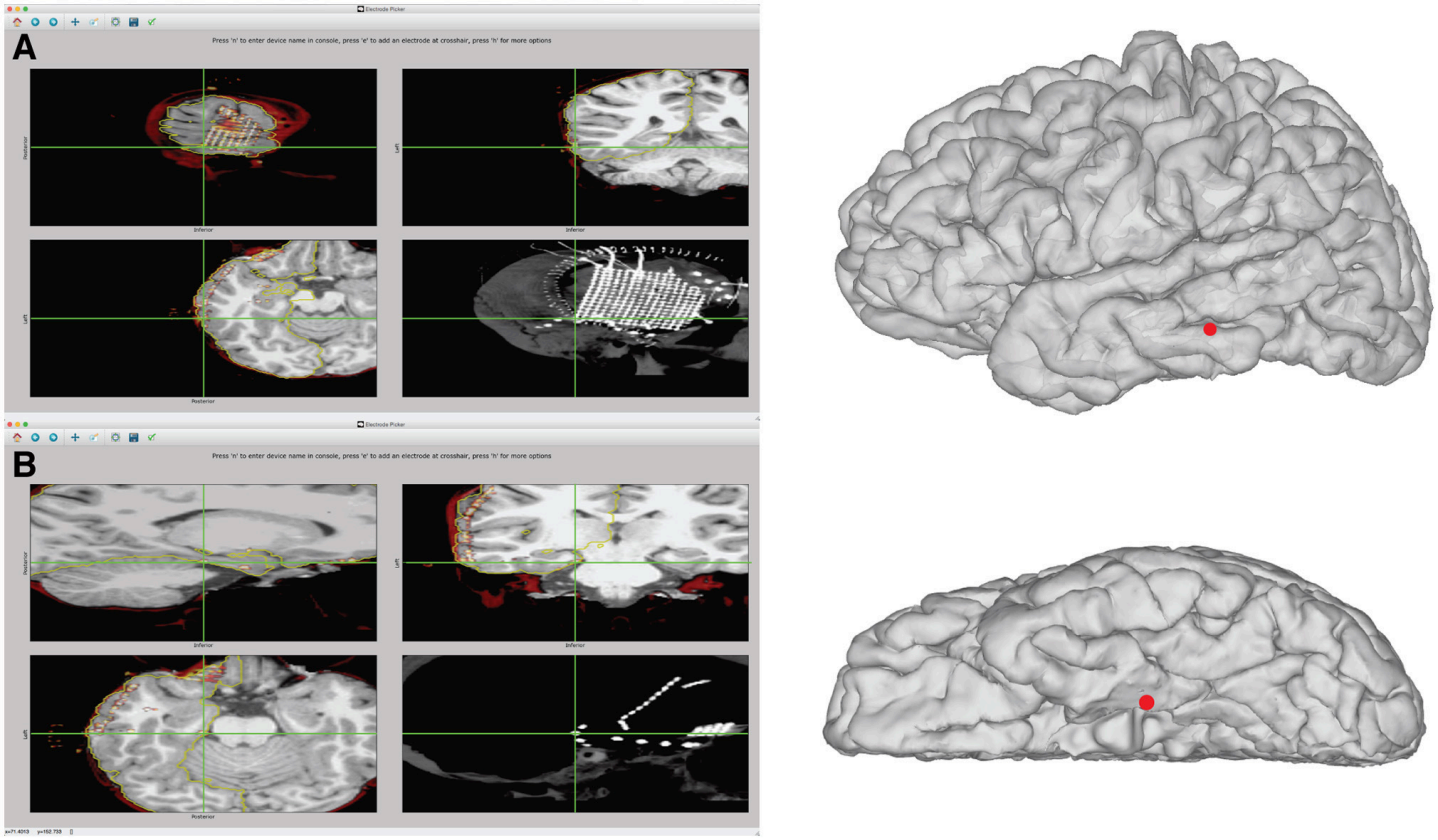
Example of identification of electrode coordinates using electrode picker. (A) Demonstrates the process of picking the coordinate for the most posterior inferior grid corner. On the left, the GUI is shown with the electrode selected. The pial surface, rCT, and skull stripped MRI are displayed. The upper left shows the electrode selected in the sagittal view. The upper right shows the coronal view. The bottom left shows an axial view. The lower right displays the intensity projection map of the CT, which is useful for visualizing the entire grid. To save the coordinate, press “n” to name a new device. With the center of the electrode artifact localized by the crosshairs in the axial, sagittal, and coronal views, press “e” to add a point. The coordinates are automatically saved to the “elecs” folder. The location of these points in 3D can be viewed by launching a separate 3D viewer by pressing “3.” This plot can be seen in the right panel. If the coordinates appear buried in the Mesh due to post-operative brain shift, additional steps can be taken to project the electrode to the surface, shown in Figure 8. (B) Example of identification of an electrode that is part of a subtemporal strip. The strip can be seen in the rCT.nii intensity projection map in the lower right panel. The coordinate is recorded from the center of the electrode artifact, seen in sagittal, coronal, and axial views. This coordinate can then be visualized on the 3D surface mesh, seen in the right panel, by typing “3.”
